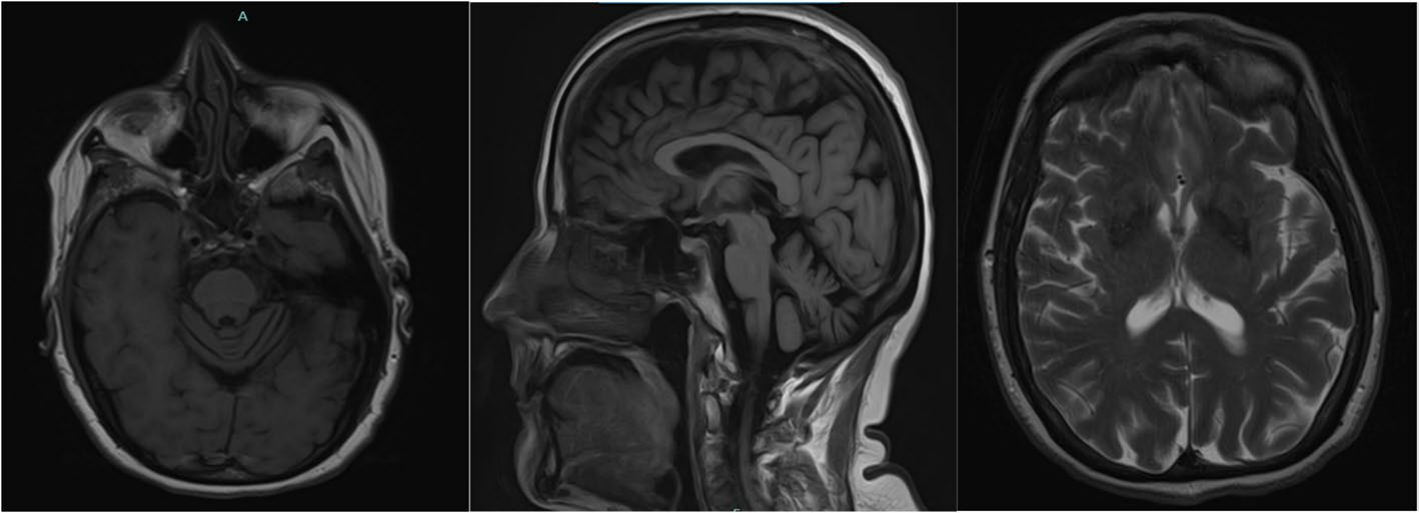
MRI brain imaging two years after symptomatic onset. Left to right–T1 axial view; T1 sagittal view; T2 axial view
